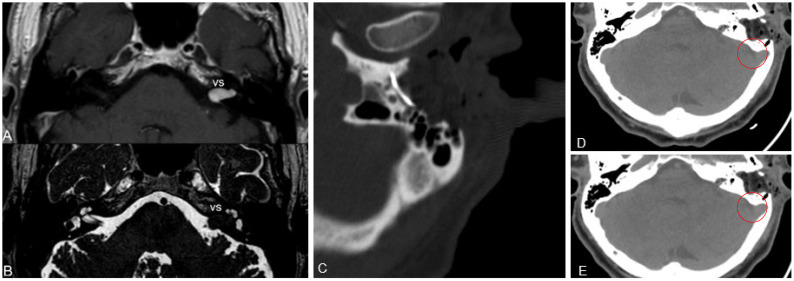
Preoperative and postoperative imaging of patient No. 1. (A) Axial gadolinium enhancement T1—weighted MRI. (B) T2—weighted drive HR MRI. (C) Postoperative CT—scan that shows the correct positioning of the array into the cochlea. (D) Suspected focal dilatation of the subarachnoid space (red circle). (E) Finding unchanged on follow-up CT scan (red circle).
